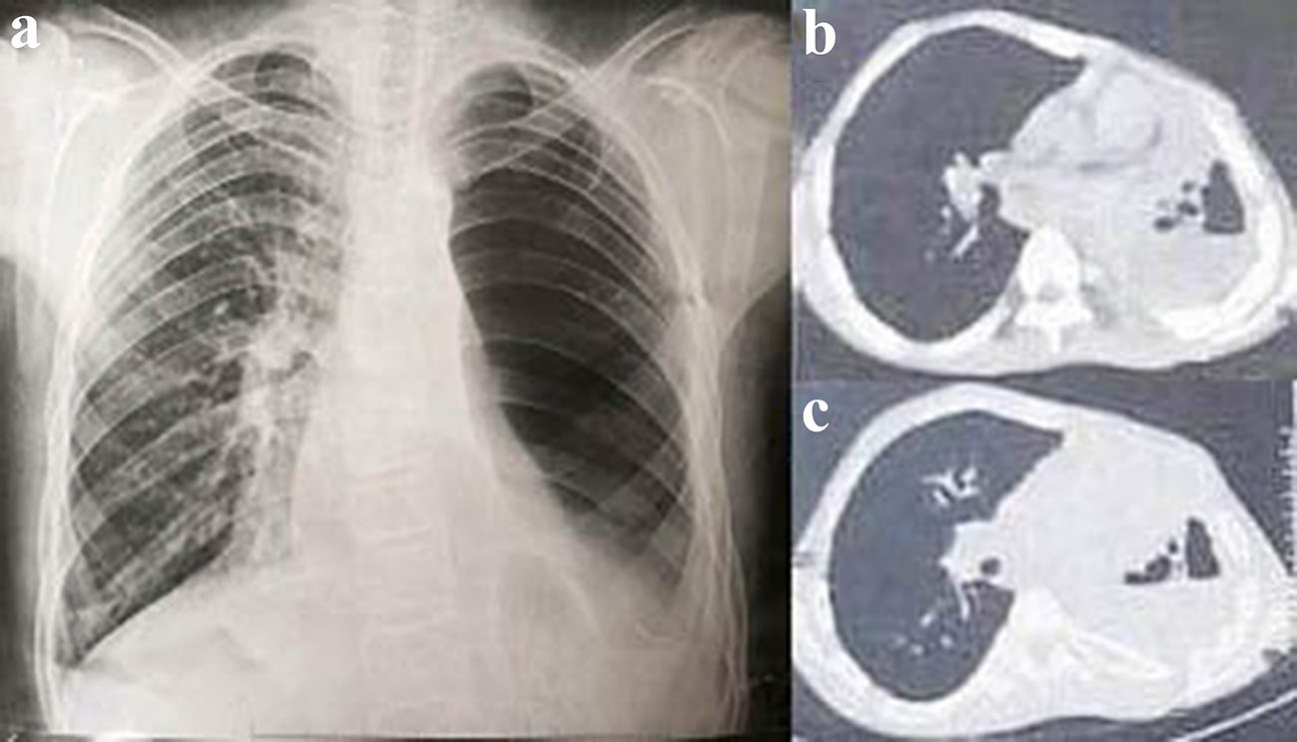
(a) Chest radiography shows gas filling the left hemithorax after pneumonectomy just after the surgery, (b) and (c) mediastinal and lung window of CT chest shows partial gas filling the left hemithorax one year after the surgery
